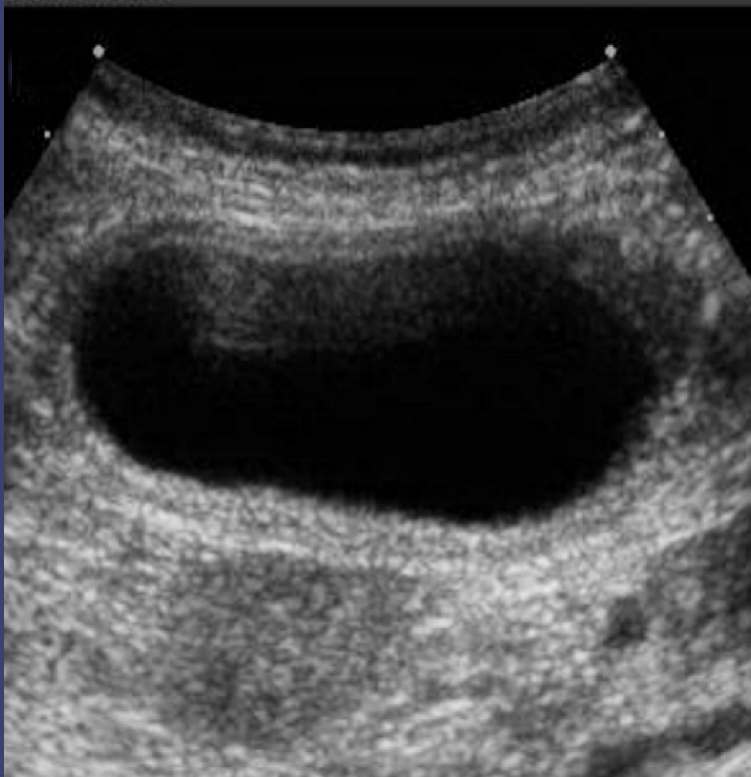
The "floating gallbladder." Ultrasound image in Patient 2 of a markedly enlarged gallbladder with a multi-layered hypoechoic rim demonstrating an edematous wall without calculi - the so-called classic description
